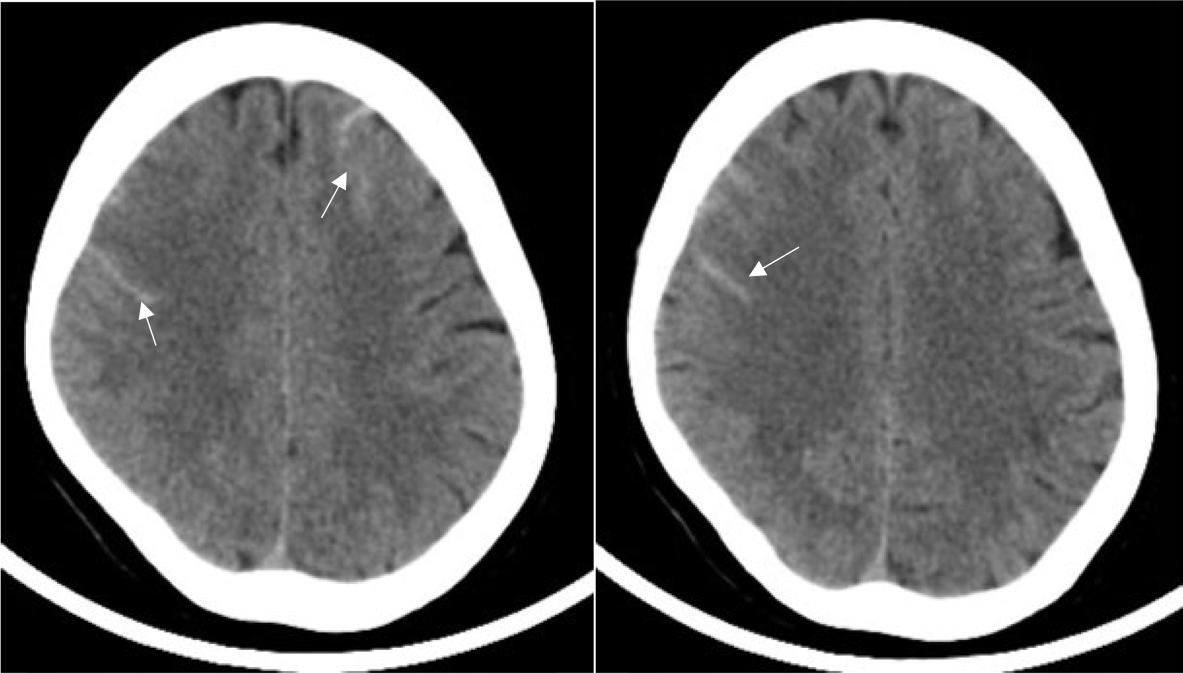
Brain CT scan in axial view. White arrows showing convexity subarachnoid hemorrhage
